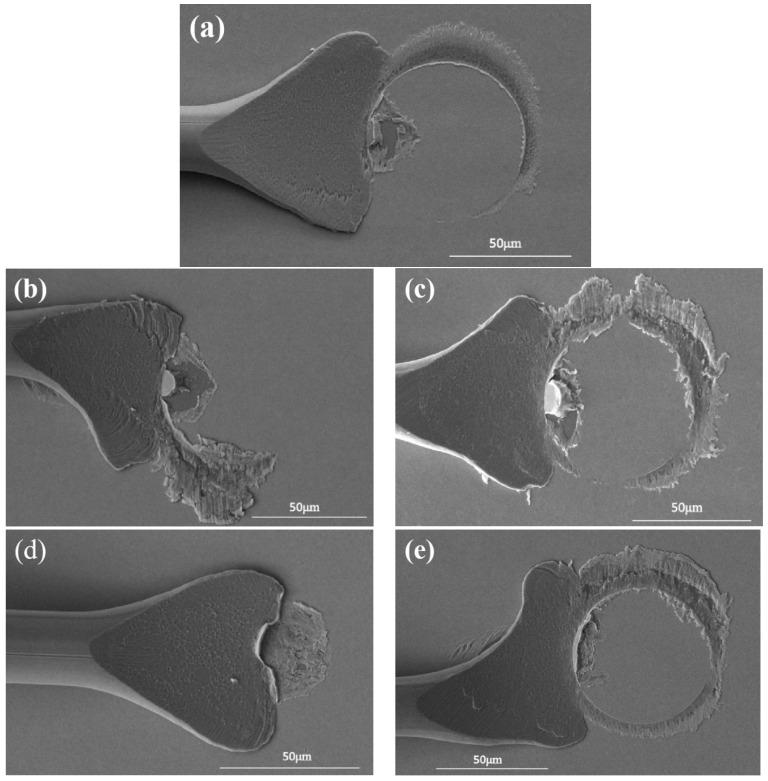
Morphology of Pd100 wedge-bonded points under different bonding pressures/ultrasonic power ((a) 50 g/110 mW; (b) 50 g/100 mW; (c) 50 g/120 mW; (d) 45 g/110 mW; (e) 55 g/110 mW).
